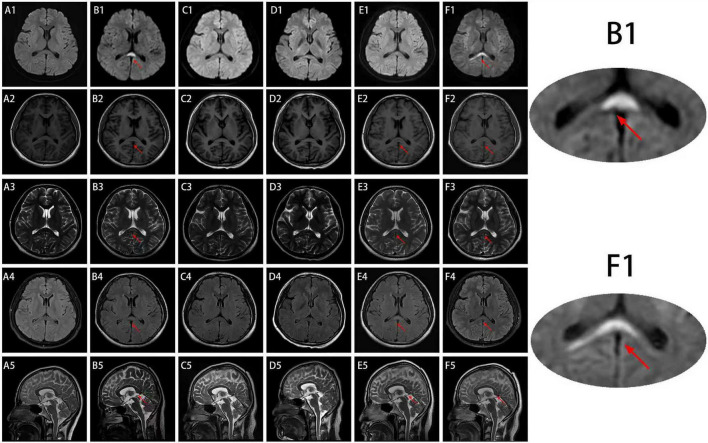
Brain Magnetic Resonance Imaging (MRI) findings of the patient during five episodes. (A2–5) No significant hyperintensity on brain MRI on day 8 after the first episode. (B1–5) Brain MRI on day 5 after the second episode showing hyperintensity (red arrow). (C1–5) The abnormal signal disappeared after 1 month of re-examination. (D1–5) No significant abnormalities on brain MRI on day 4 after the third episode. (E1) No significant abnormalities on DWI on day 4 after the fourth episode; T1WI, T2WI, and FLAIR (E2–5) on day 7 showing hyperintensity in the splenium of the corpus callosum. (F1–5) Abnormal signals in the splenium of the corpus callosum on day 4, (A1) the abnormal signal disappeared after 8 months. [(A1–F1): DWI, (A2–F2): T1WI; (A3–F3) and (A5–F5): T2WI; (A4–F4): FLAIR].
